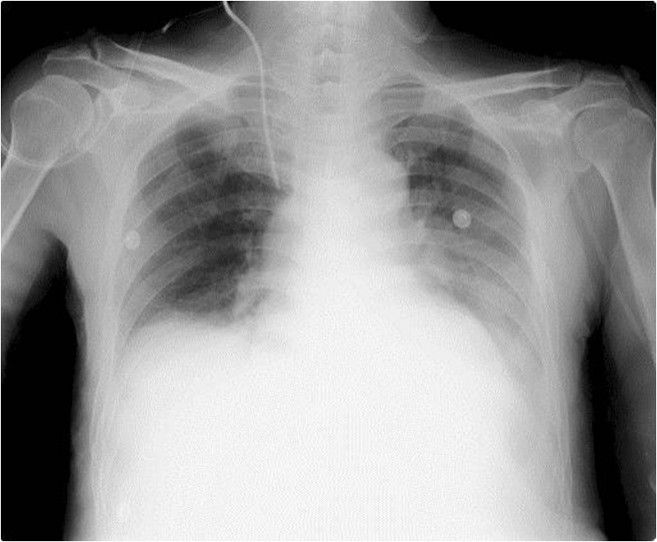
Chest X-ray on POD 9 after NHF therapy was applied shows improvement of the atelectasis.
